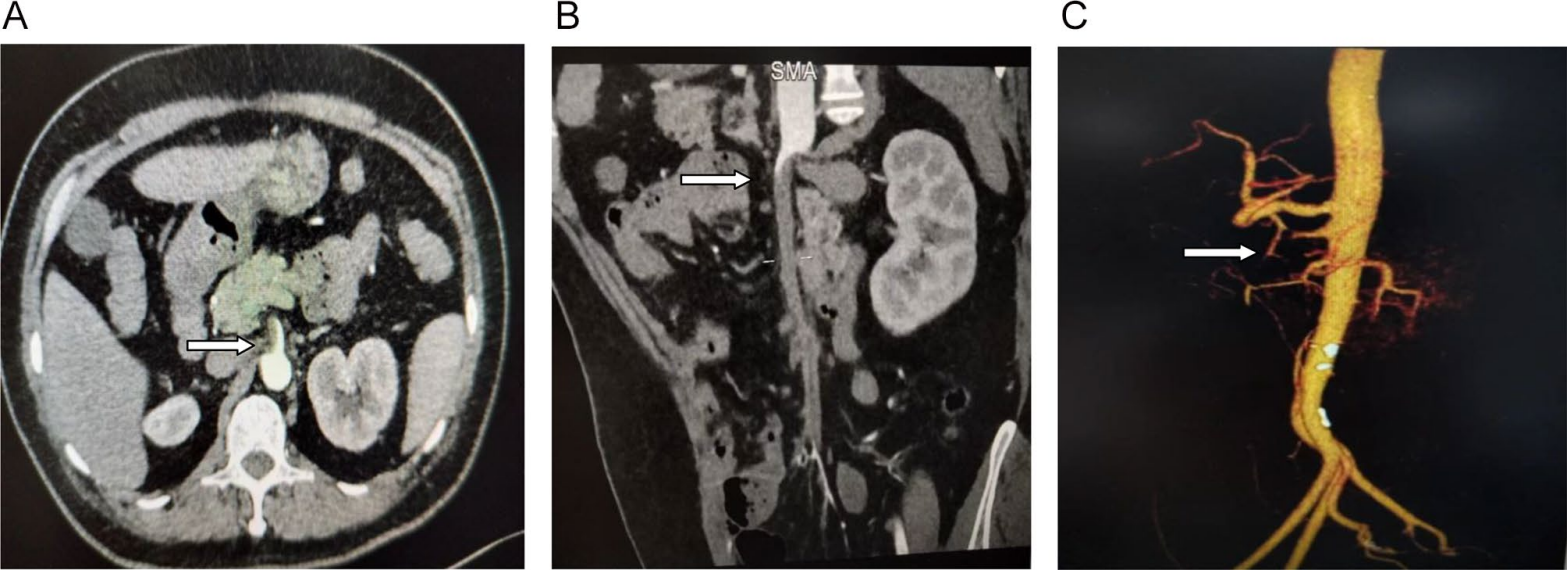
Superior mesenteric artery thrombosis in CTA (coronal (A) and sagittal (B) section). SMAT in three-dimensional reconstruction (C)
